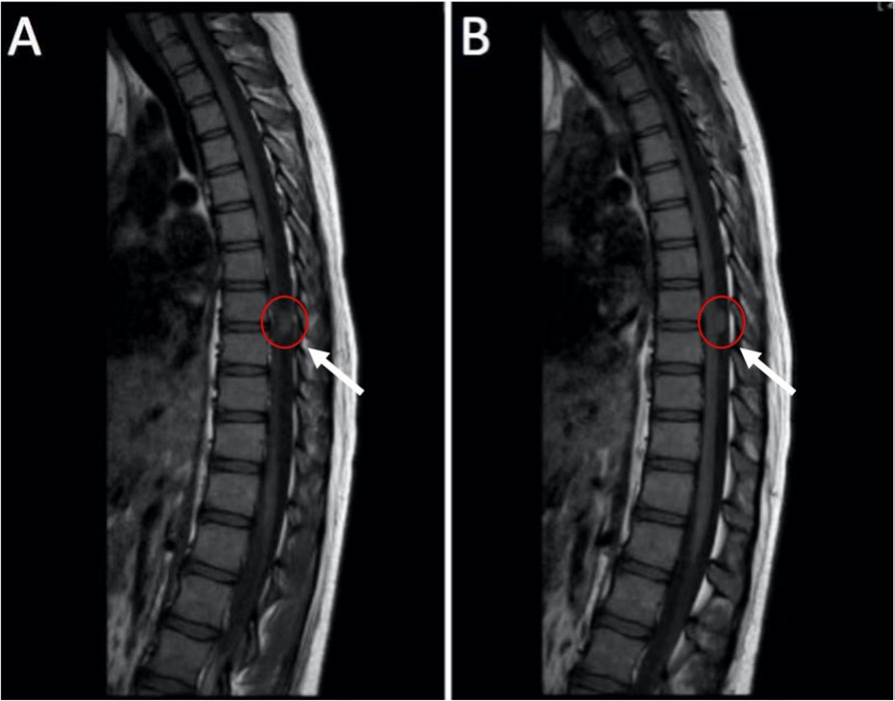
Small mass with abnormal signal at T8-9 on spinal MRI.
